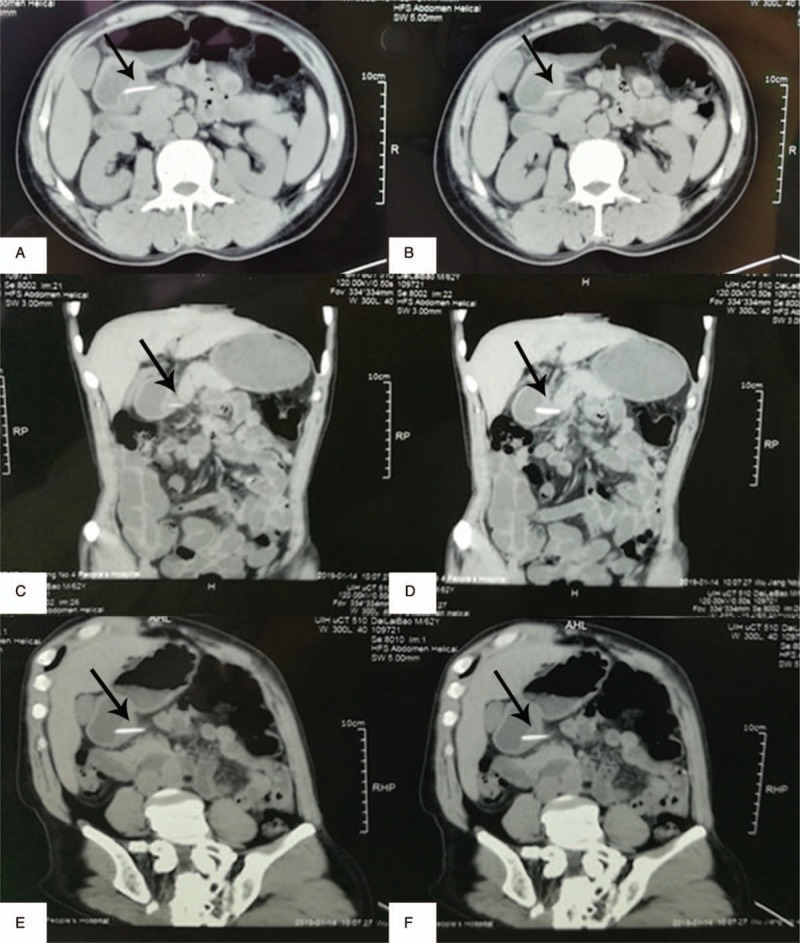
Abdominal CT showing a linear, hyperdense, foreign body (arrow) perforating the lesser gastric curvature. (A, B: Transverse section; C, D: Coronal section; E, F: Sagittal section).
